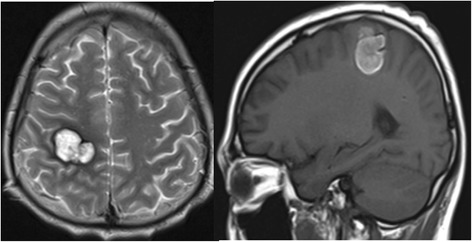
The MRI scans show a reduction in the size of multiple brain metastases within the right Parietal lobe in comparison with the prior images
